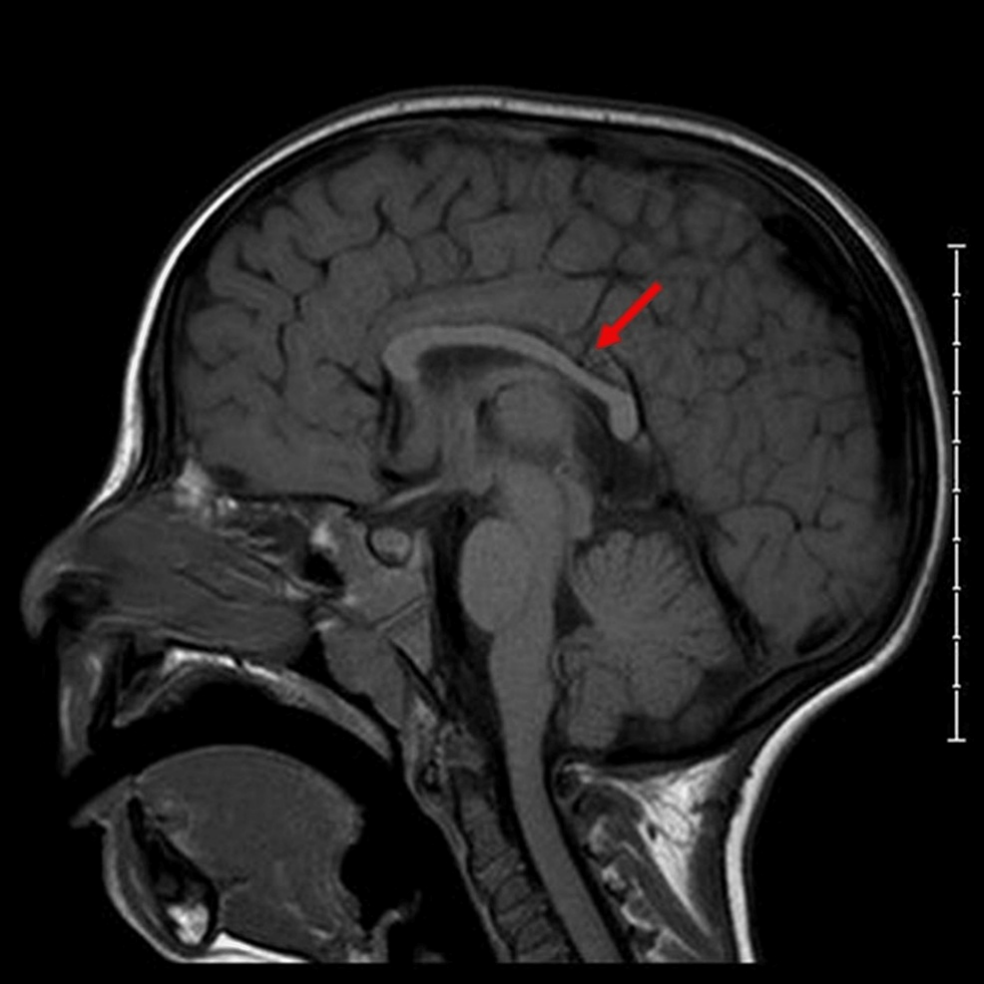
Non-contrast brain MRI. Sagittal midline T1-weighted image showing thinning of the corpus callosum (red arrow).
